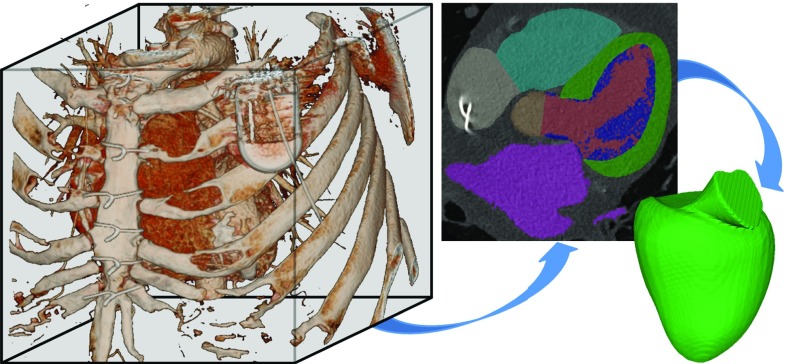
Model extraction from CT images
